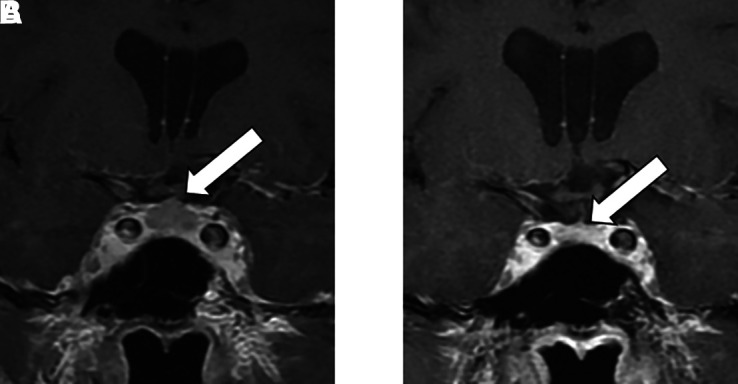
MRI imaging of the pituitary gland (white arrows) before (left) and 6 months after (right) a spinal blood patching in a patient with CSF leak (imaging corresponding to another patient with CSF leak resulting in enlargement of the pituitary gland; case and images courtesy of Dr. John L.D. Atkinson).
